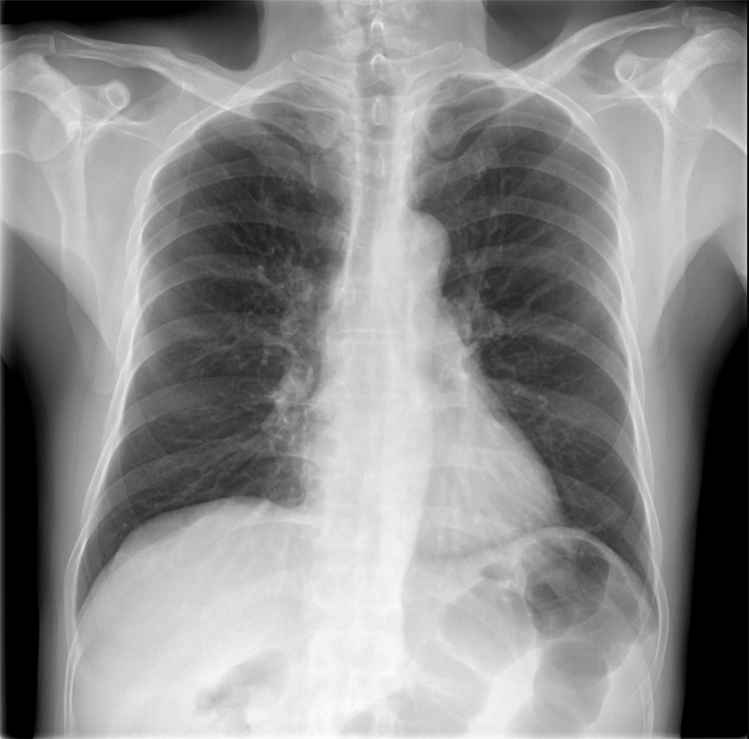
Chest radiographs show no obvious substantial lesions in both lungs.
